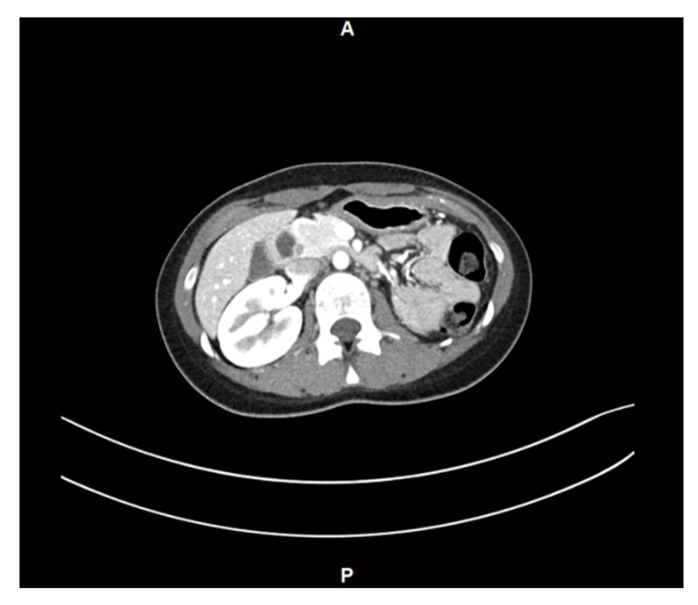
Contrast-enhanced computed tomography (CT) scan of abdomen shows the absence of left kidney.
